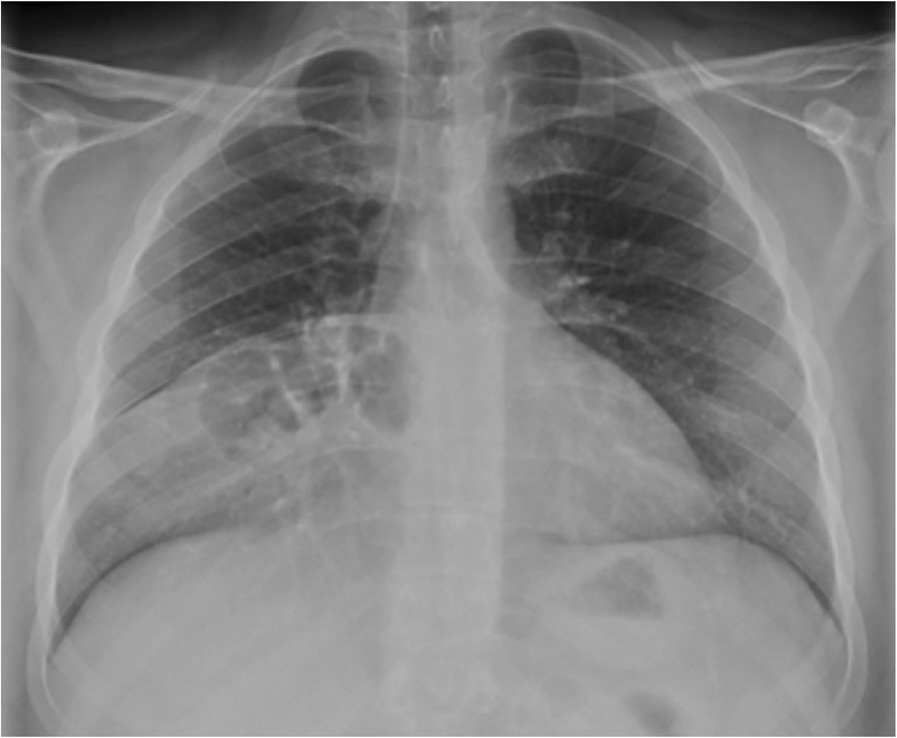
Chest x-ray showing posteroanterior view of bowel loops in the right pleural cavity
